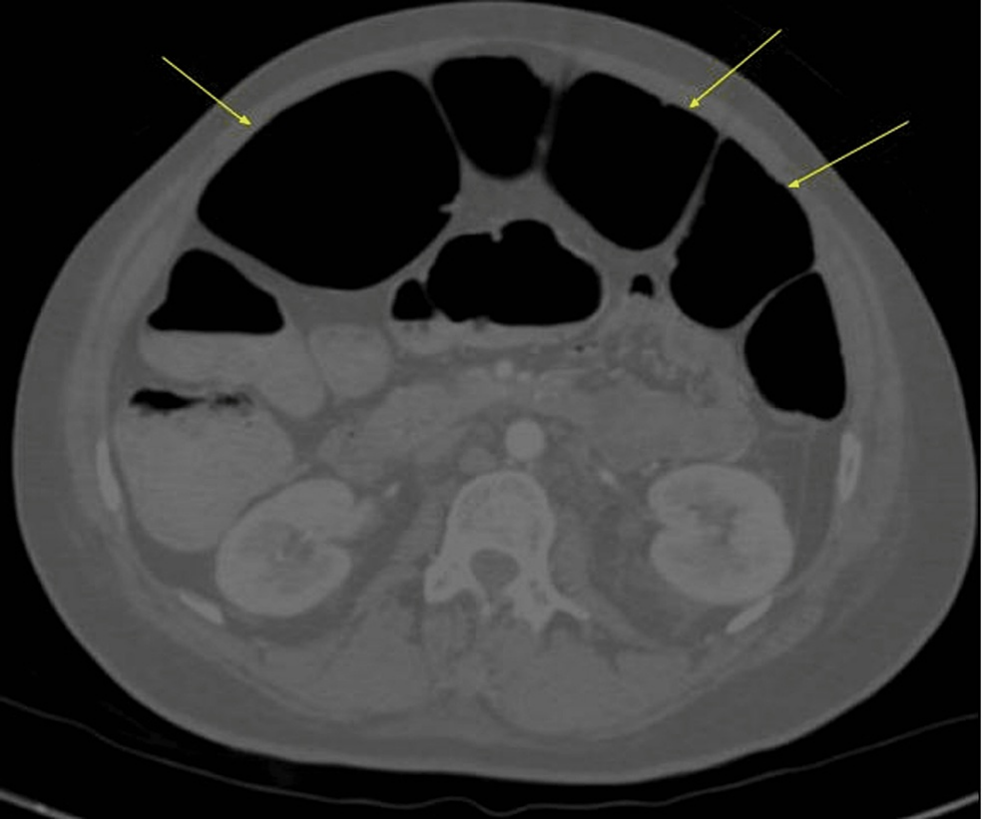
Abdominal CT scan axial image shows a maximum dilatation of 9.5 cm at the transverse colon and sigmoid (yellow arrows)
